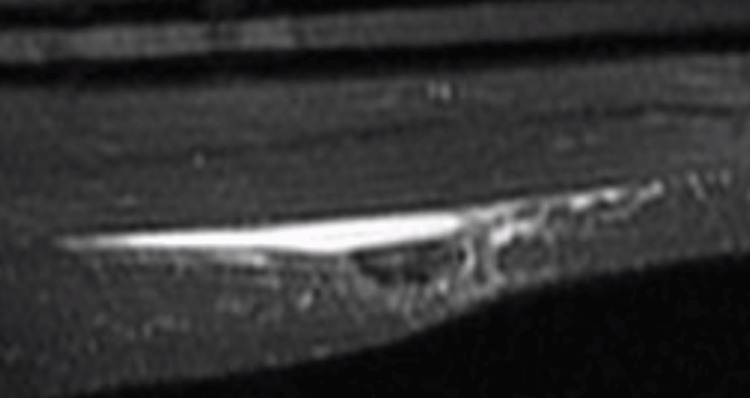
T2-weighted coronal MRI through the right thigh demonstrating fluid showing subcutaneous tissue and vastus lateralis.
